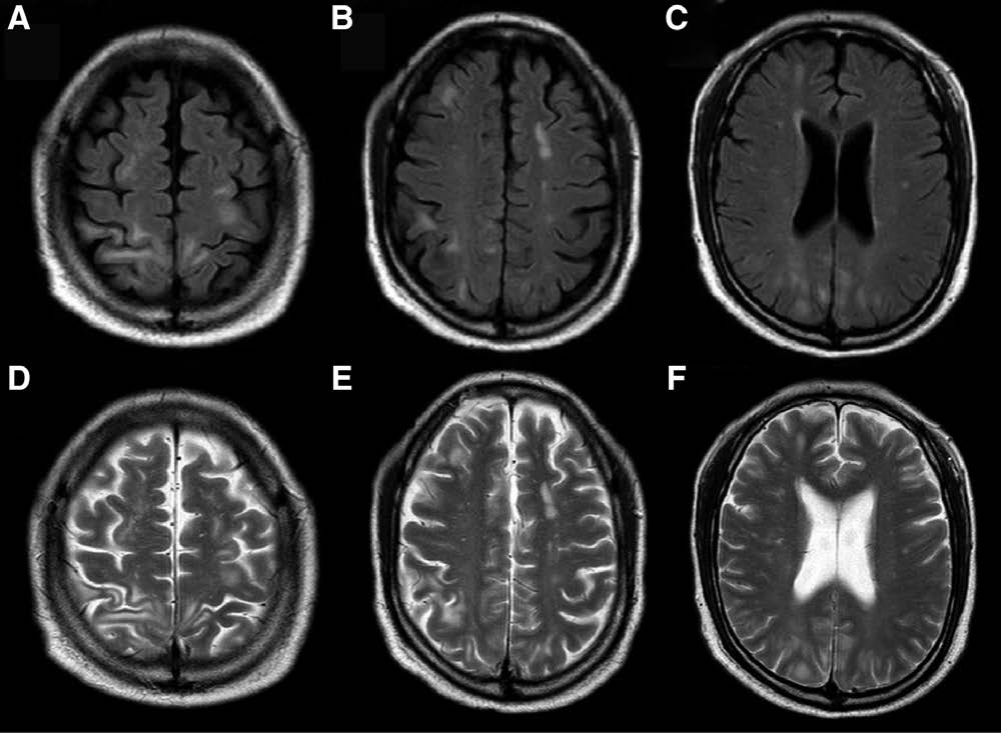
The patient’s first cranial MRI examination on the 14th day of admission (A–C) FLAIR sequence, (D–F) T2WI sequence: multiple patchy and gyriform FLAIR and T2WI hyperintense lesions in the gray matter and gray-white matter junctions of both frontal, parietal, and occipital lobes, as well as high signal intensity in T2WI. FLAIR = fluid attenuated inversion recovery, MRI = magnetic resonance imaging, T2WI = T2 weighted imaging.
